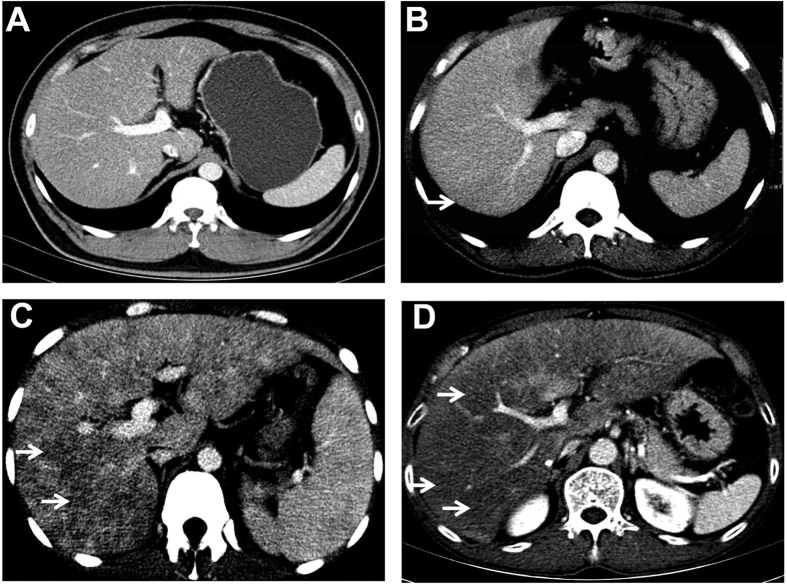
The severity of heterogeneous hypoattenuation on CT images: (A) equivocal, (B) mild, (C) moderate, and (D) severe heterogeneous hypoattenuation in the liver. Arrow: heterogeneous hypoattenuation. Because heterogeneous hypoattenuation is obvious in portal phase, the data were obtained from portal phase CT scan.
